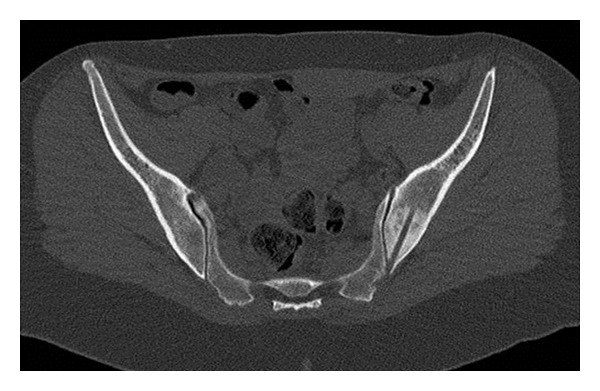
CT scan of the pelvis of a 33-year-old lady with low back pain. X-rays were normal as was an MRI of the lumbar spine. A bone scan showed increased activity in the ilium adjacent to the sacroiliac joint, and a CT showed a dense sclerotic lesion. This would have been typical for fibrous dysplasia radiologically, but this did not explain the pain. CT-guided needle biopsy confirmed the diagnosis. This CT image clearly shows the lesion and the path of the biopsy.
